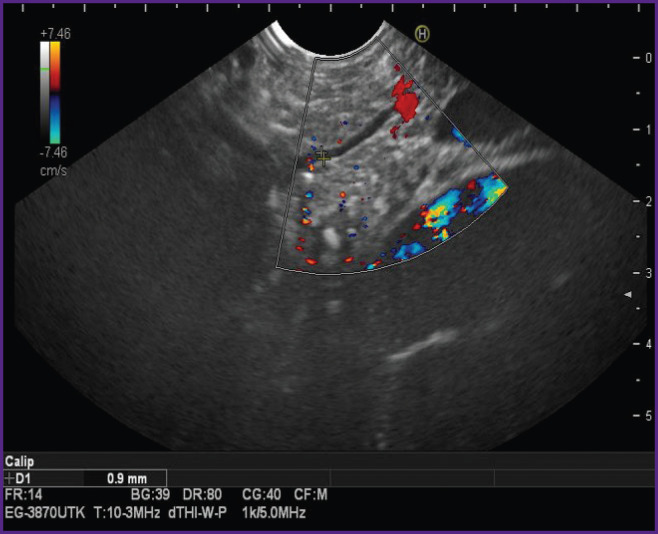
Endoscopic ultrasound examination of the pancreas: alcifications are detected in the parenchyma
